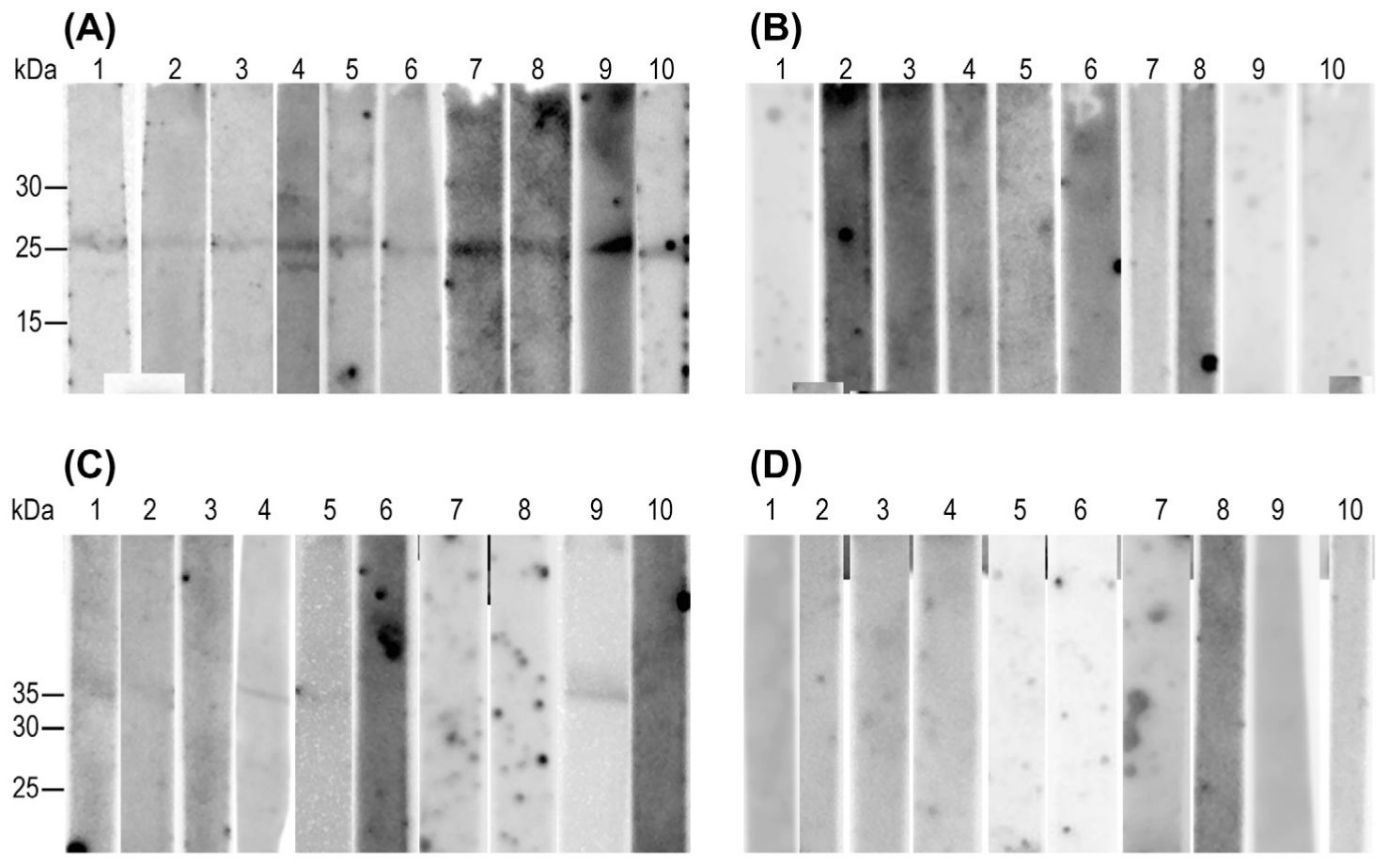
Immunoblot analysis of recombinant antigens Tsol-p27 (A and B) and cC1(C and D). (A and C) Probing done with sera (diluted 1:500) from patients diagnosed as NCC positive by CT (lanes 1–4). (B and D) Probing done with sera (diluted 1:500) from patients diagnosed as NCC negative by CT.
